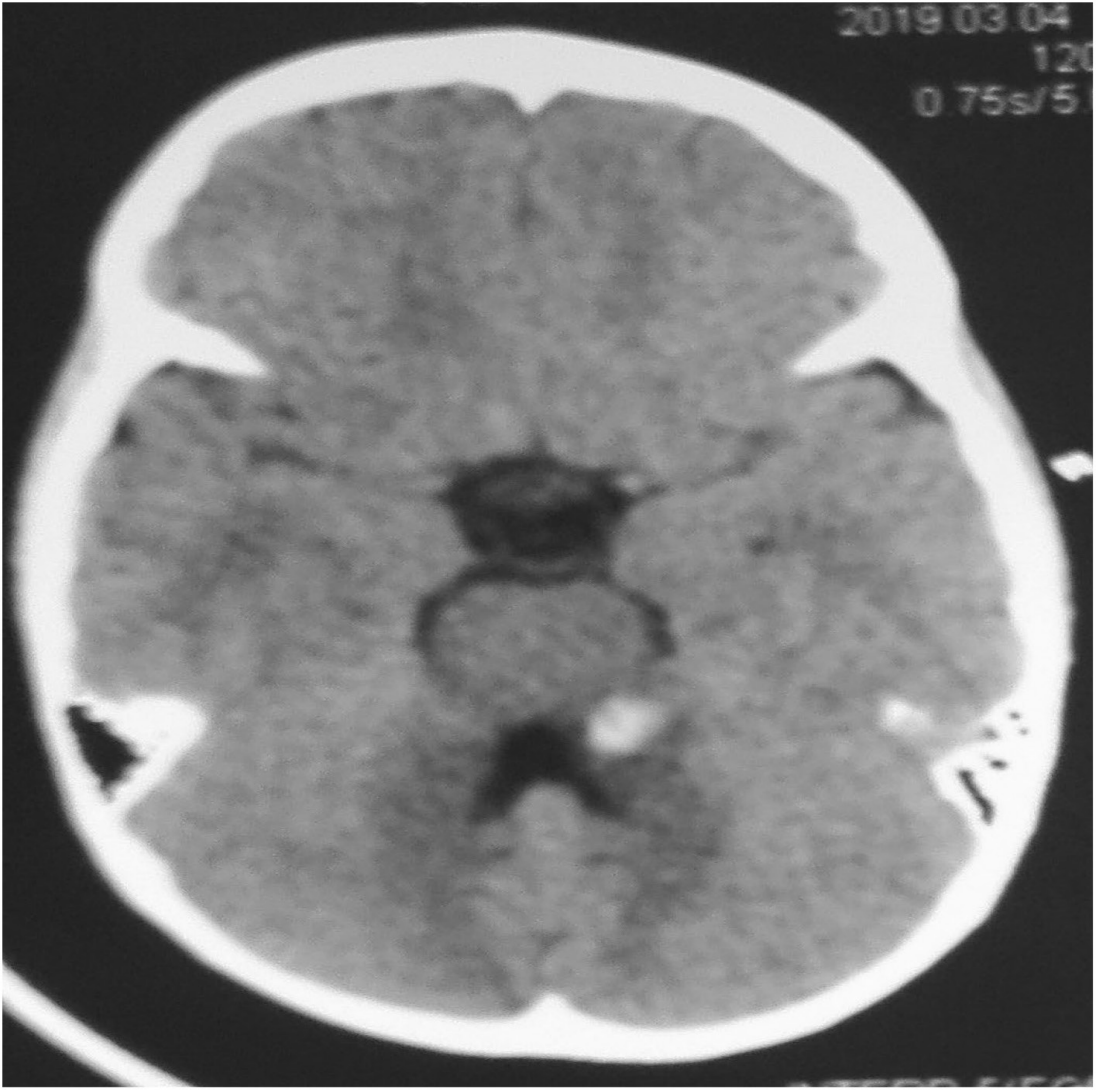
Non‐contrast CT brain axial slice performed at the initial presentation. The CT shows hyperdense lesion (resembling hemorrhage/hematoma) seen in the region of the left superior peduncle and dentate nucleus. The reminder of the scan was unremarkable.
